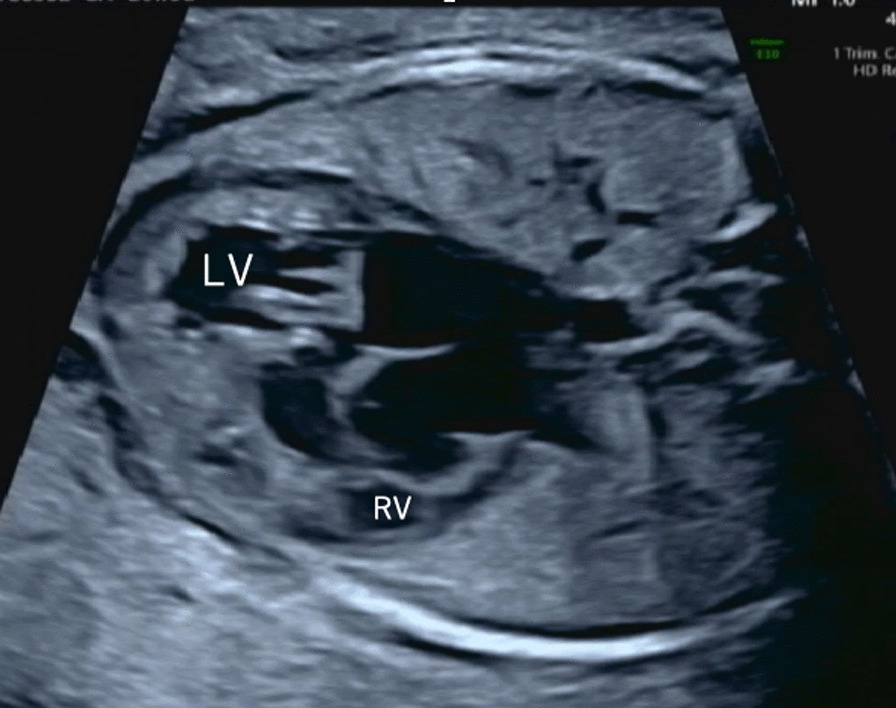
Fetal ultrasound images; 26 weeks and 5 days of gestation in the third pregnancy. The CTAR was 50% and there was pericardial effusion, thoracoabdominal effusion, decreased left ventricular contractility, and a decreased cardiovascular profile score of 4 points
